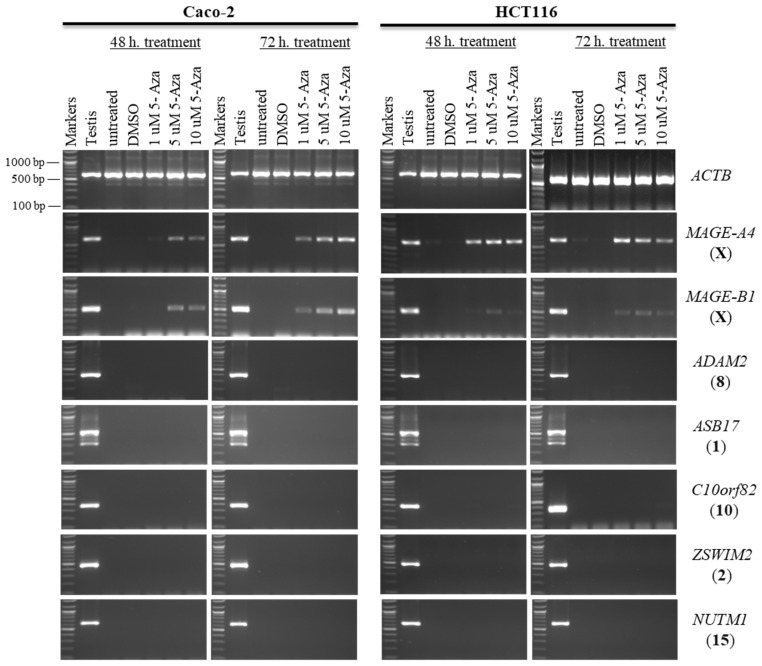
The effects of 5-aza-CdR treatments on testis-specific gene expression profiles in the Caco-2 and HCT116 cancer cell lines. The expression of *ADAM2*, *ASB17*, *C10orf82*, *ZSWIM2*, and *NUTM1* genes is shown on agarose gels following treatment with various doses of 5-aza-CdR for 48 h (left column of each cell) or 72 h (right column of each cell). Untreated Caco-2 and HCT116 cells were used as controls to compare the expression of CT genes with treated cells, and a testis sample served as a positive control for primer efficiency. The control Caco-2 and HCT116 cells were treated with DMSO, as DMSO was the solvent used in the 5-aza-CdR solution. The positive control for the cDNA samples is the expression of the *ACTB* gene. The official names of the genes are written to the right of the agarose gel images, and the location of each gene on the chromosomal is written in parentheses on the right. Above each lane, the particular concentration of 5-aza-CdR (1, 5, and 10 µM) is written.
